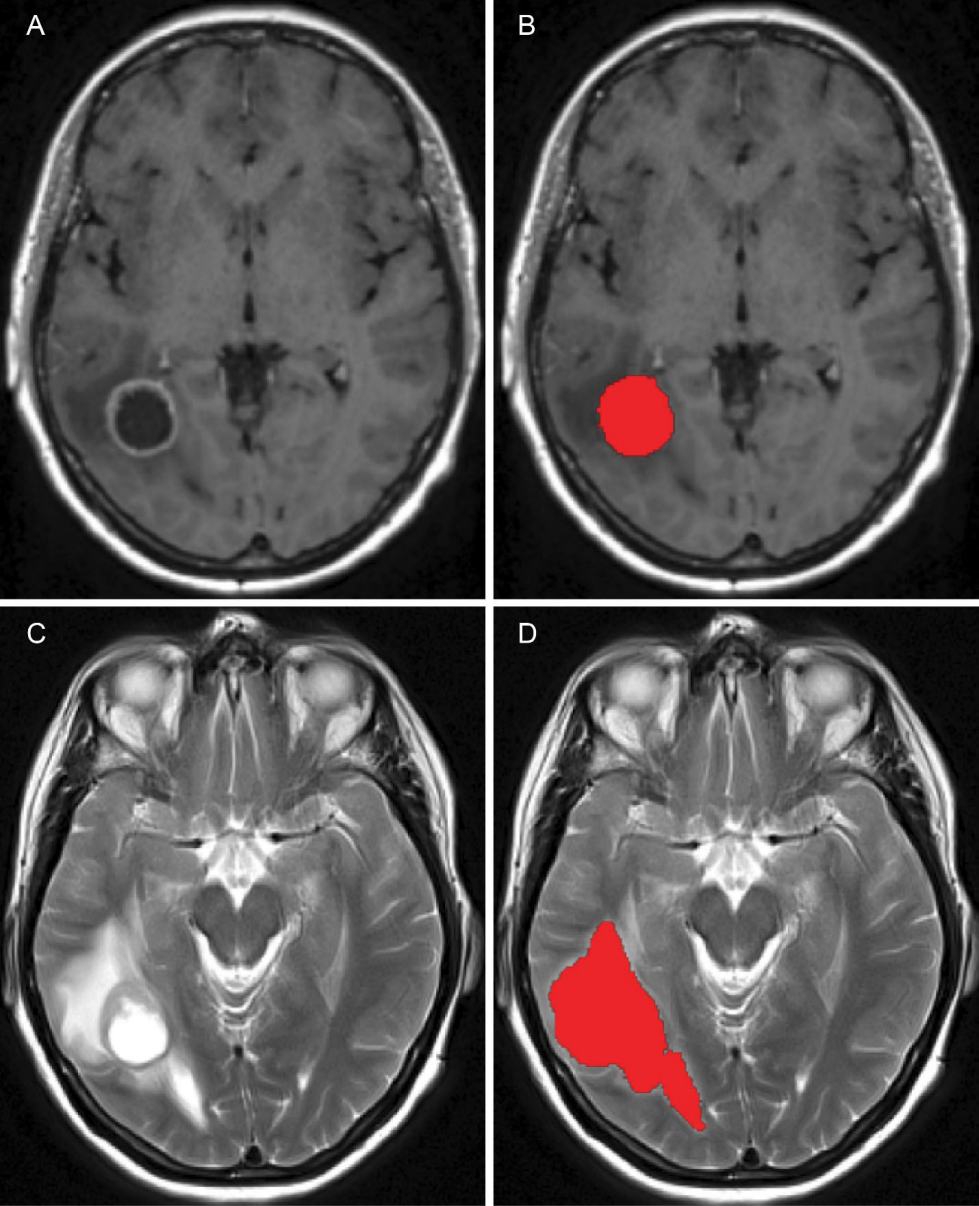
Figure showing the pre–surgical axial MRI scans and corresponding volumes of interest for the patient in question: (A) T1+C scan, (B) Pre–surgical GBM VOI, (C) T2 scan and (D) oedema VOI.
